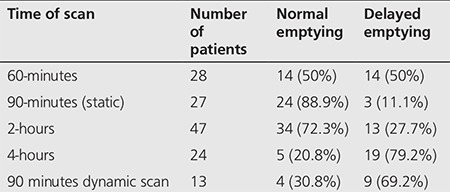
Patient data at five time points of gastric emptying scintigraphy
